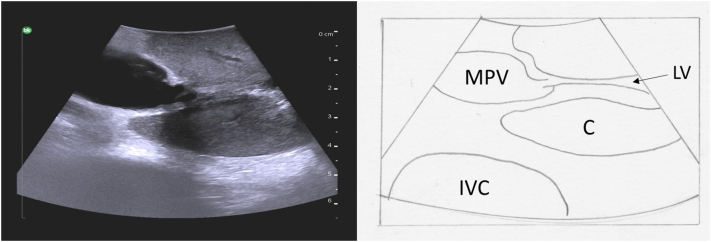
Intraoperative ultrasound with a transverse view of the caudate lobe. MPV main portal vein, IVC inferior vena cava, C caudate, LV ligamentum venosum.
